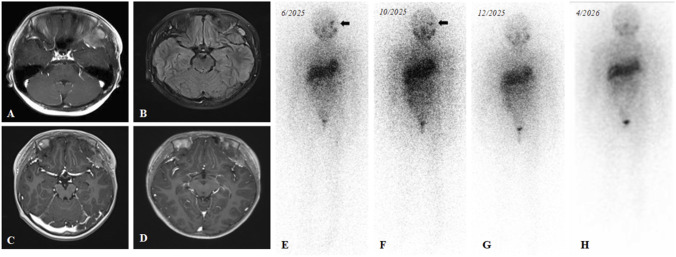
Imaging findings of Patient 2. Black arrows indicate the dominant soft-tissue lesion in the left temporal region. (A) MRI at the time of second relapse in July 2025; the local soft-tissue lesion measured 27.7 mm × 23.0 mm × 15.6 mm. (B) MRI after salvage ICE chemotherapy in August 2025. (C) MRI after two cycles of combination therapy in October 2025; the local soft-tissue lesion measured 24.4 mm × 17.6 mm × 8.2 mm. (D) MRI after four cycles of combination therapy in December 2025. (E) MIBG imaging at second relapse in June 2025. (F) MIBG imaging after two cycles of combination therapy in October 2025. (G) MIBG imaging in December 2025. (H) MIBG imaging in April 2026. No residual abnormal MIBG uptake was detected on images G and H, with complete regression of the previous metastatic lesion.
